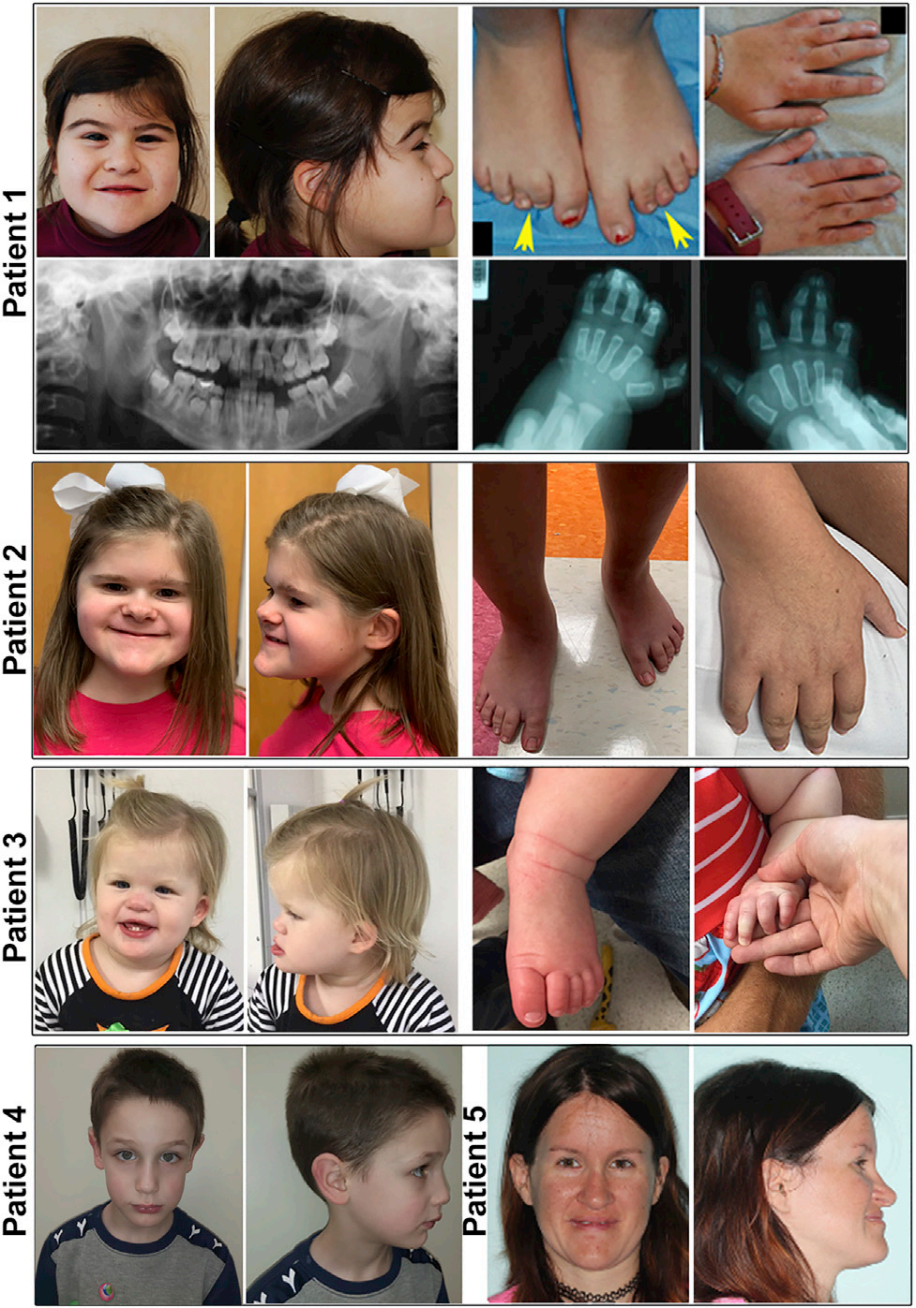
Clinical presentation of patients with *CSNK2B* variants Front and side views of subjects 1–3 diagnosed with IDCS show strikingly similar facial gestalt, whereas subjects 4 and 5 diagnosed with POBINDS show vivid difference of facial gestalt, and no obvious digital or limb anomaly was recorded as in IDCS. Digital anomalies of IDCS patients are shown in two panels on right side. For subject 1, arrows show brachydactyly 2–5 and syndactyly 2–3 of the digits of the feet. Notably, preoperative radiographs of the hands and teeth are shown for subject 1.
